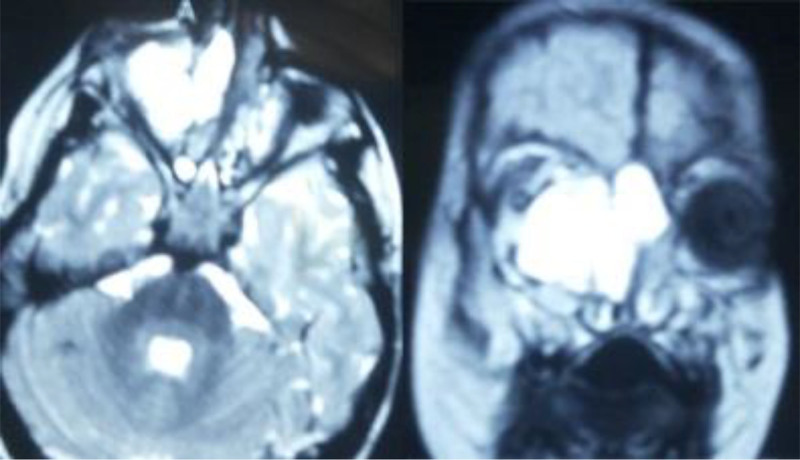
MRI T2-weighted imaging revealed a hyperintense lesion located in the periorbital area and the anterior skull base.
